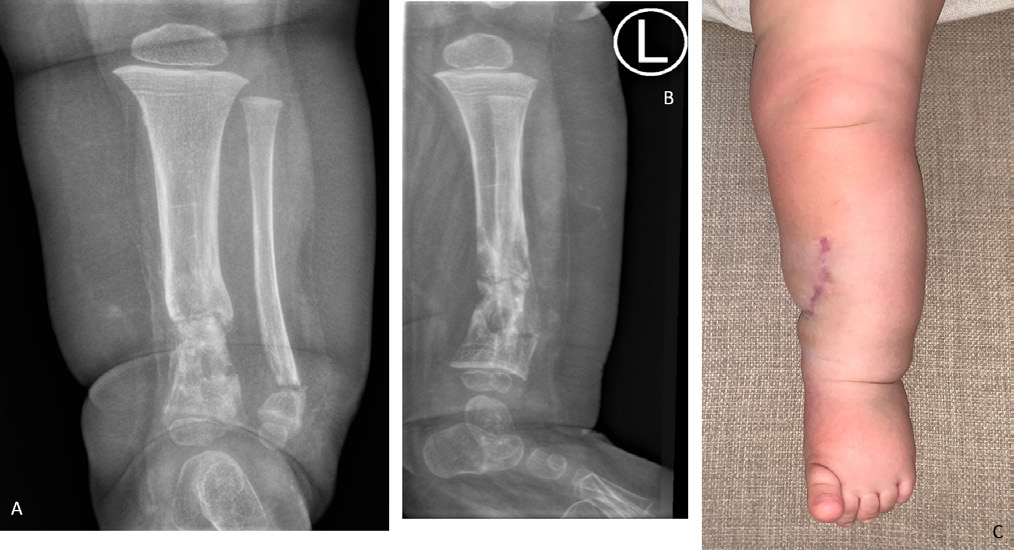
Anteroposterior (A) and lateral radiographs (B) of the affected limb (aged 14 months). There is post-surgical new bone formation with defined cortices of the distal tibia and fibula, and reactive sclerosis at the osteotomy sites. The focal lesion has a non-aggressive radiological appearance and the proximal diaphyses remain spared. (C): Clinical photograph demonstrating orthogonal alignment of the limb and minimal visual deformity as compared with Figure 1
